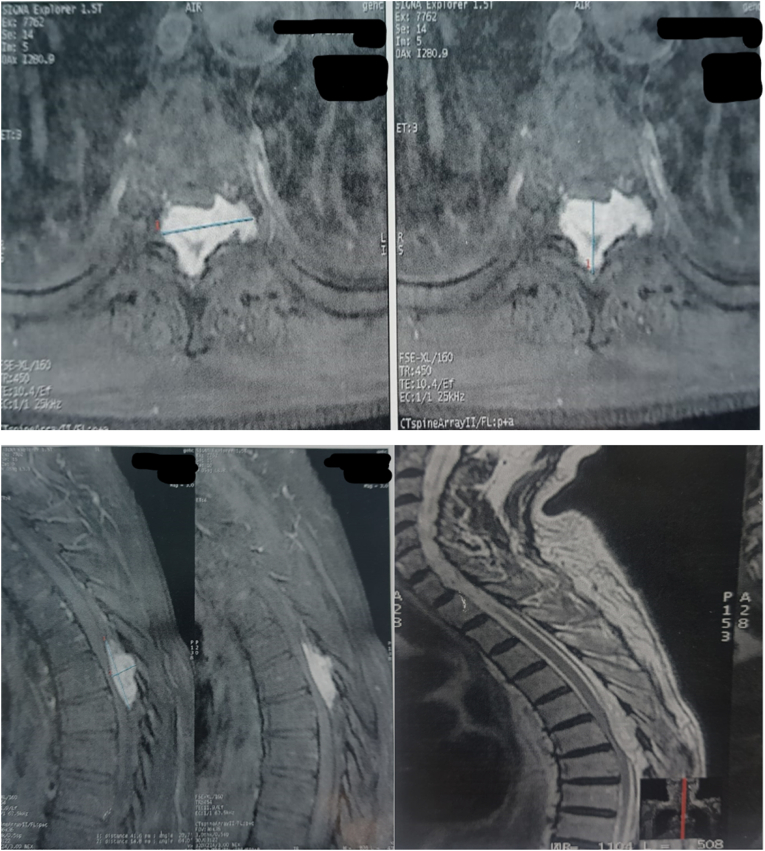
Spinal cord MRI revealed a tissue-like process at the level of T6-T7, intradural, intermediate T1 signal and T2 hypersignal and strongly enhanced after gadolinium injection with left foraminal extension measuring 25 mm in width and 13.5 mm in anteroposterior diameter and extending over a height of 30 mm.
